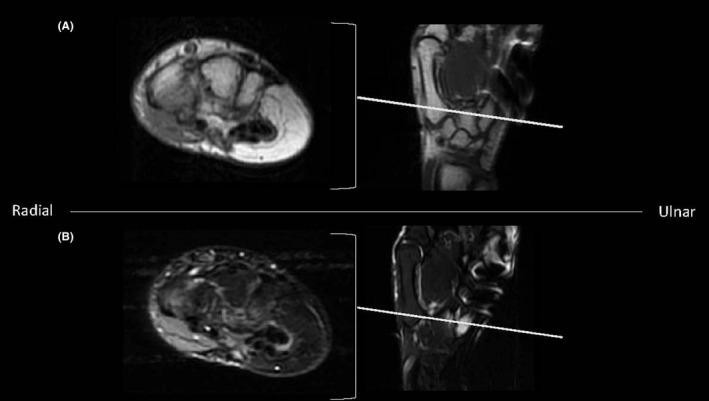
14‐month postoperative MRI (without contrast) showing (A) T1 and (B) T2 images of the left hand
